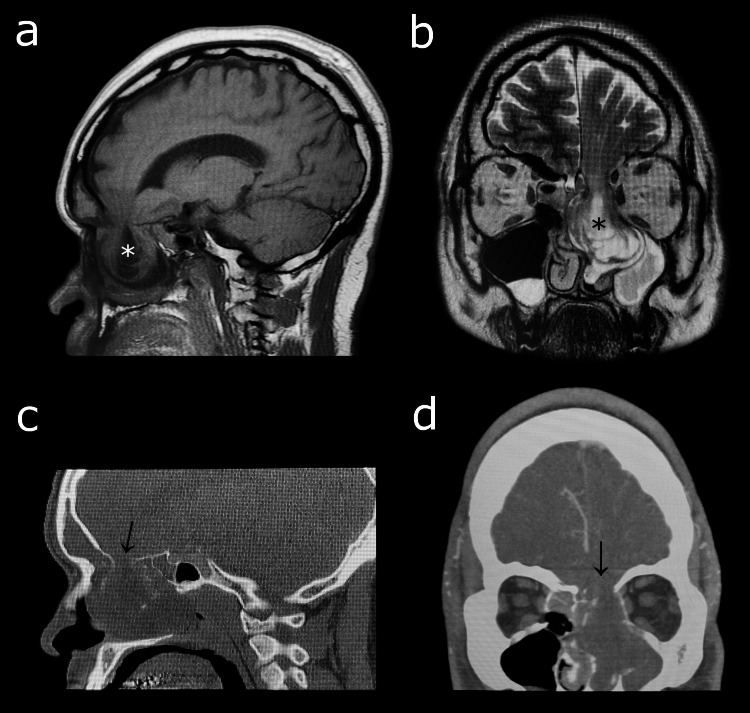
Imaging findings. A 43-year-old male presented with a lifelong history of headache, blurry vision, anosmia, and rhinorrhea. CT and MRI showed a 36 × 35 × 33 mm encephalocele herniating into the left ethmoids and nasal fossa with bony remodeling. (a) Sagittal T1 MRI and (b) coronal T2 MRI showing the encephalocele (*). (c) Sagittal CT and (d) coronal CT showing the bony defect (arrows). The encephalocele was resected and the cribriform plate was reconstructed. The patient was discharged with no recurrence of symptoms. CT: computed tomography; MRI: magnetic resonance imaging
